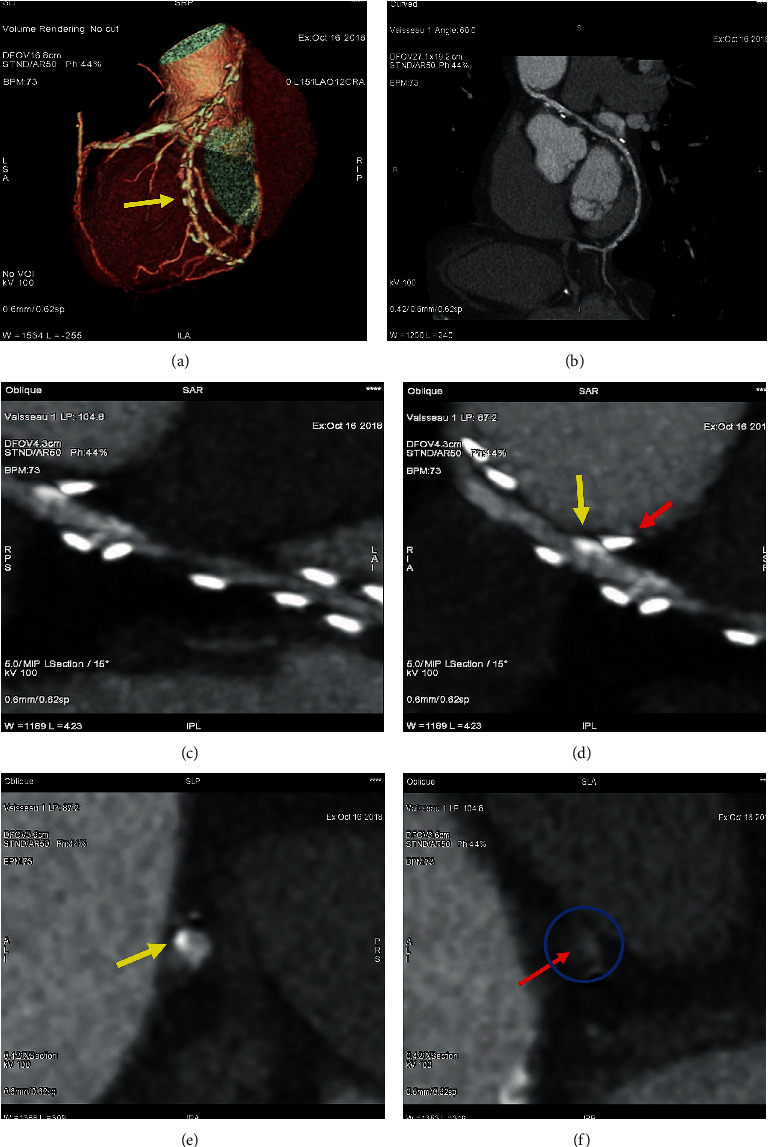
(a–f) CT angiographic control of RA used as second target conduit on second obtuse branch of LCC and PDA. (a) Volume rendering imaging of RA (yellow arrow) running at the level of Theil sinus transversus. (b–f) 2D curved imaging. (b) Course of the radial artery; (c) stenosis of the body of the RA graft. (d) Red arrow shows the metallic clip while yellow arrow highlights a fibrous plaque that are contiguous. (e) Yellow arrow shows a calcific plaque of RA conduit. (f) Note in the blue circle the stenosis of RA due to a fibrous plaque; red arrow shows a remodeling plaque. (LCC2). RA: radial artery; LCC: left coronary circumflex; LCC1: first obtuse branch; LCC2: second obtuse branch; RCA: right coronary artery.
